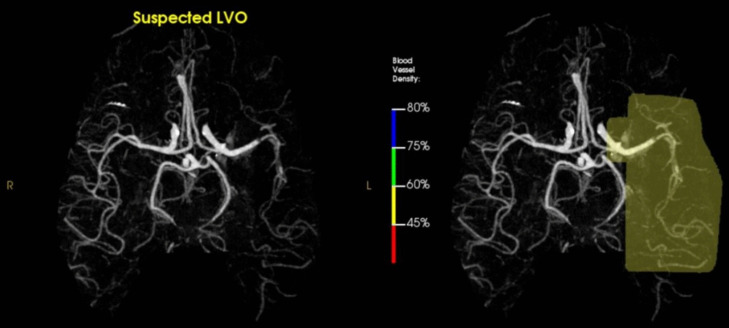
Reconstructed 3D images of an intracranial computed tomography (CT) angiography demonstrating RAPID-software detecting large vessel occlusion
